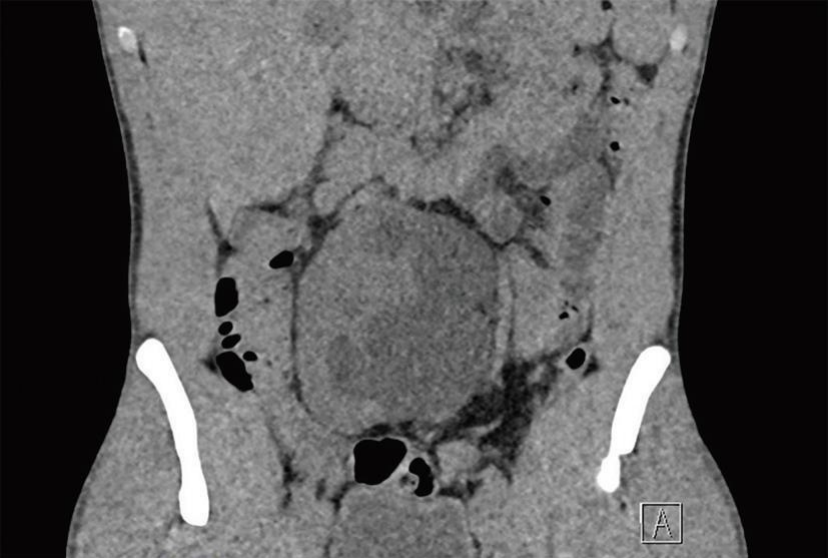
CT-abdomen (native low dose) showcasing ectopic malrotated pelvic right kidney with largely intrarenal renal pelvis and accompanying hydronephrosis.
